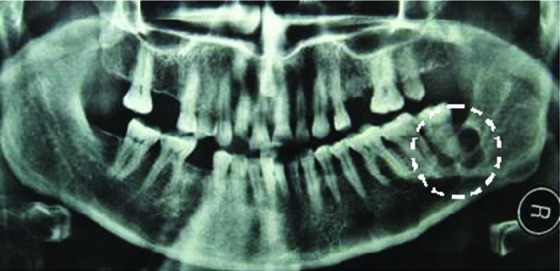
Panoramic view: a soap bubble multilocular radiolucency with well-defined margins in mesial, distal and periapical of third molar (white circle).
